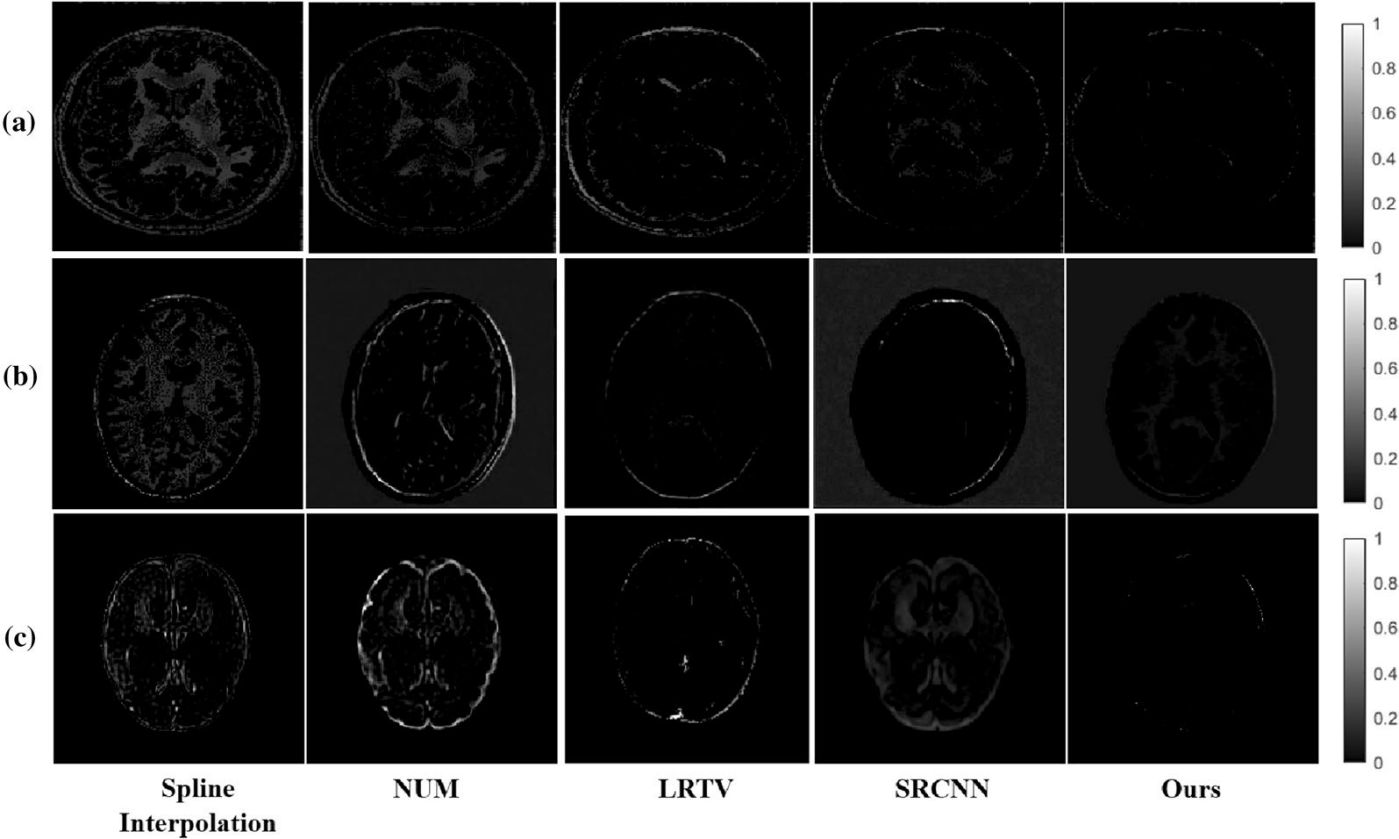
The error maps of SR results: (a) Kirby 21; (b) NAMIC; (c) clinical fetal brain MR images.
